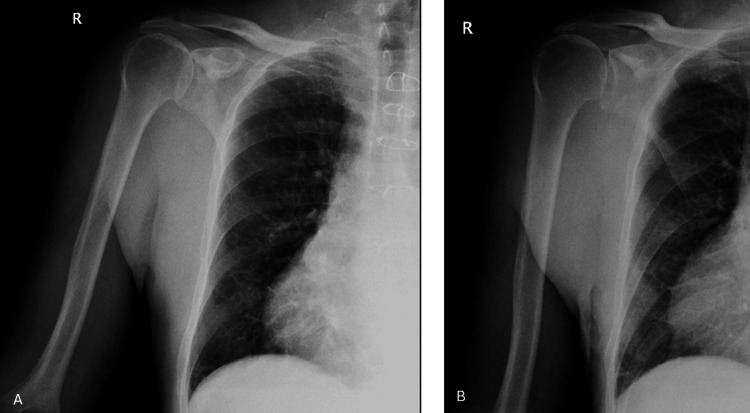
X-ray images of the patient’s right shoulder taken upon arrival. (A) Anteroposterior (AP) view of the shoulder; (B) True AP view of the shoulder.
